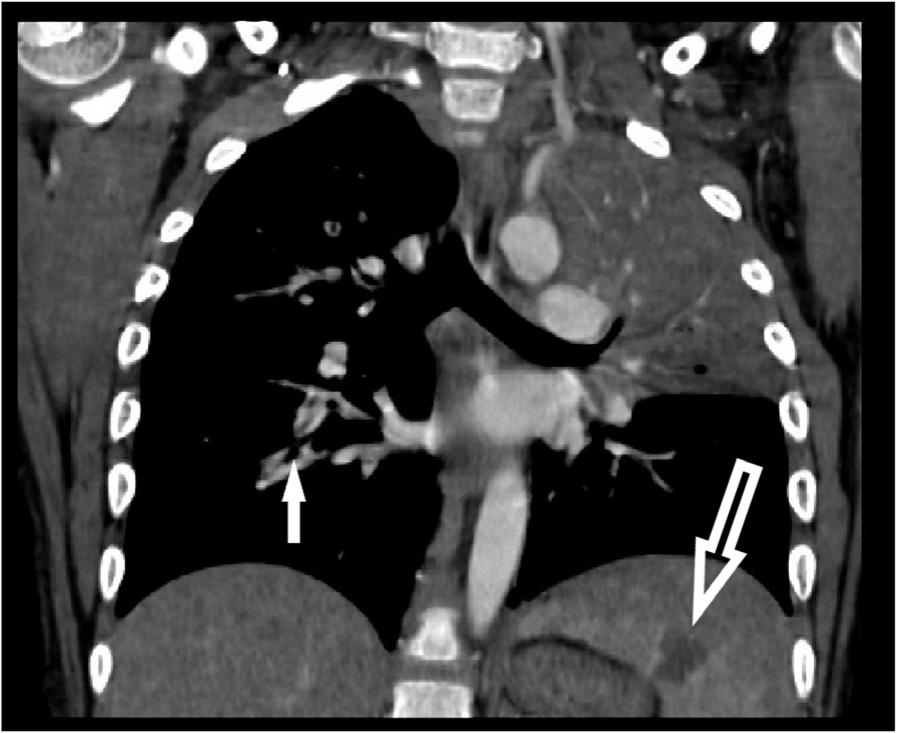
CT of chest, coronal view, showing consolidation in Left upper lobe of lung, large splenic infarct (large open arrow), and filling defect of a Right pulmonary embolus (small solid arrow)
